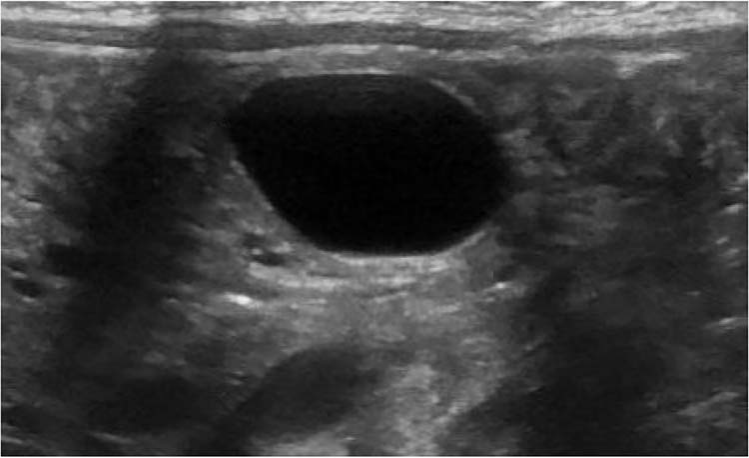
Ultrasound with enteric cyst with gut signature.
